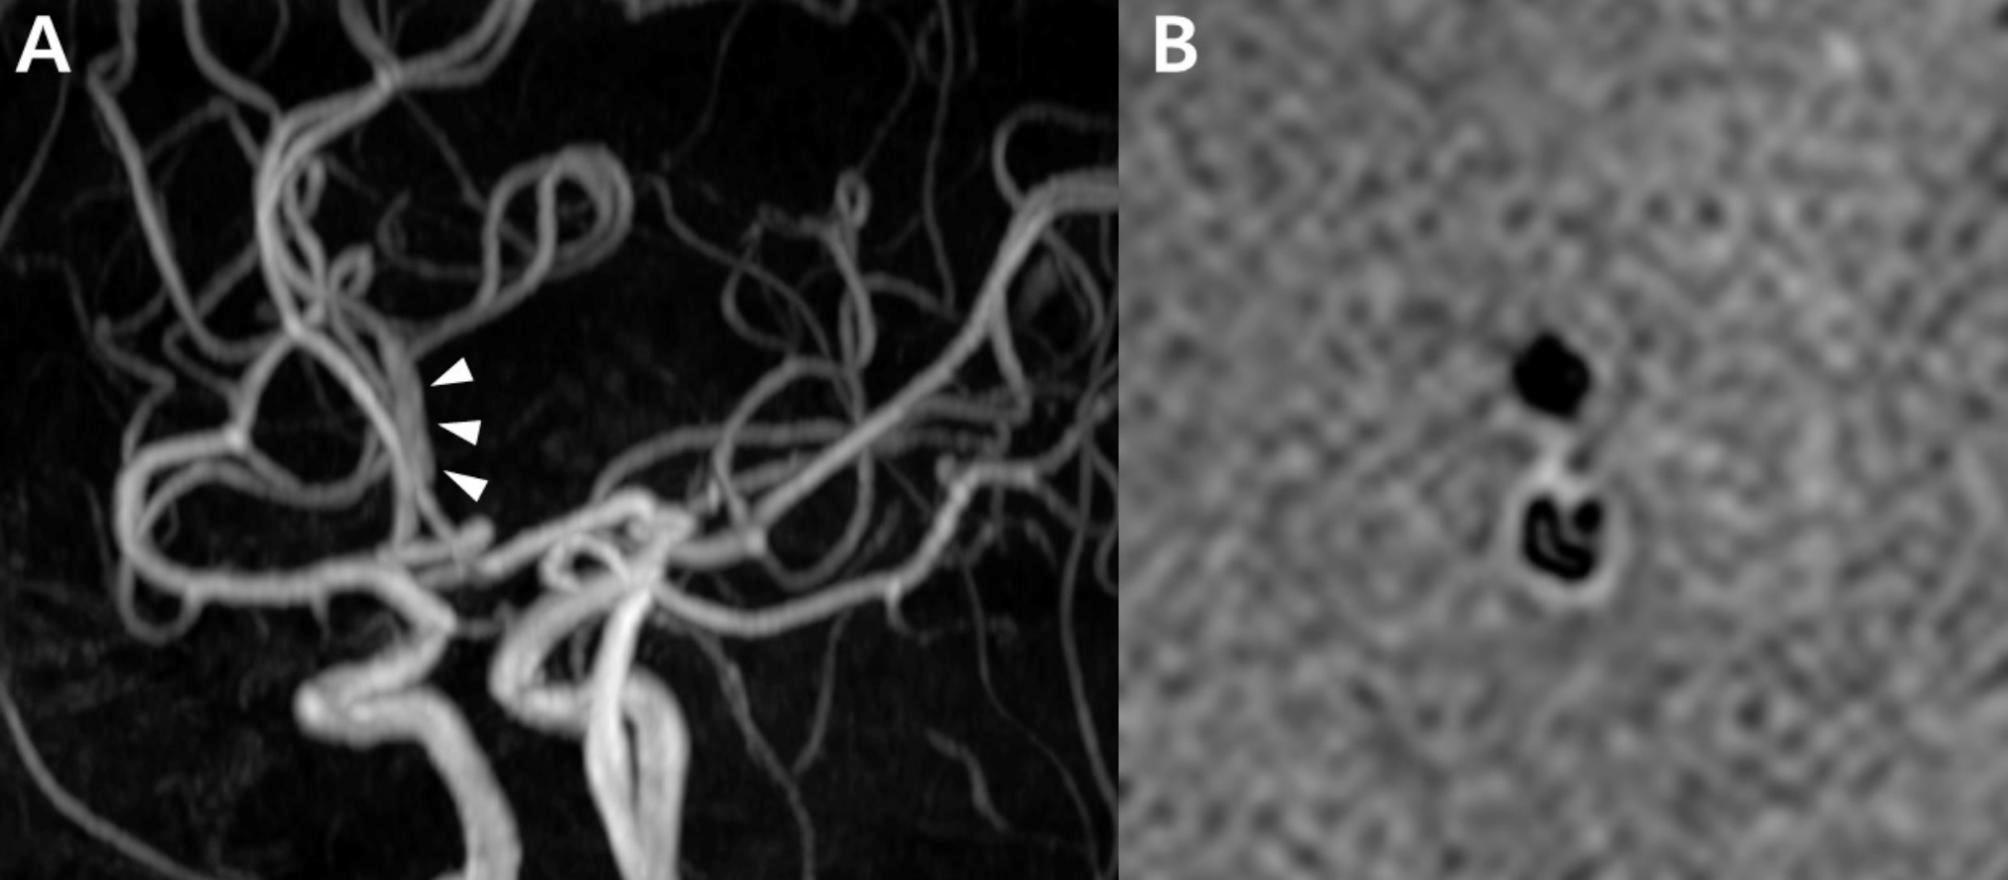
Mimickers of reversible cerebral vasoconstriction syndrome: Intracranial arterial dissection (case 9). The MRA shows a single dilation (arrowhead) in the left A2 segment in a patient with thunderclap headache (Case 9), who is ultimately diagnosed with dissection by (B) high-resolution vessel wall MRI revealing a dissecting flap
